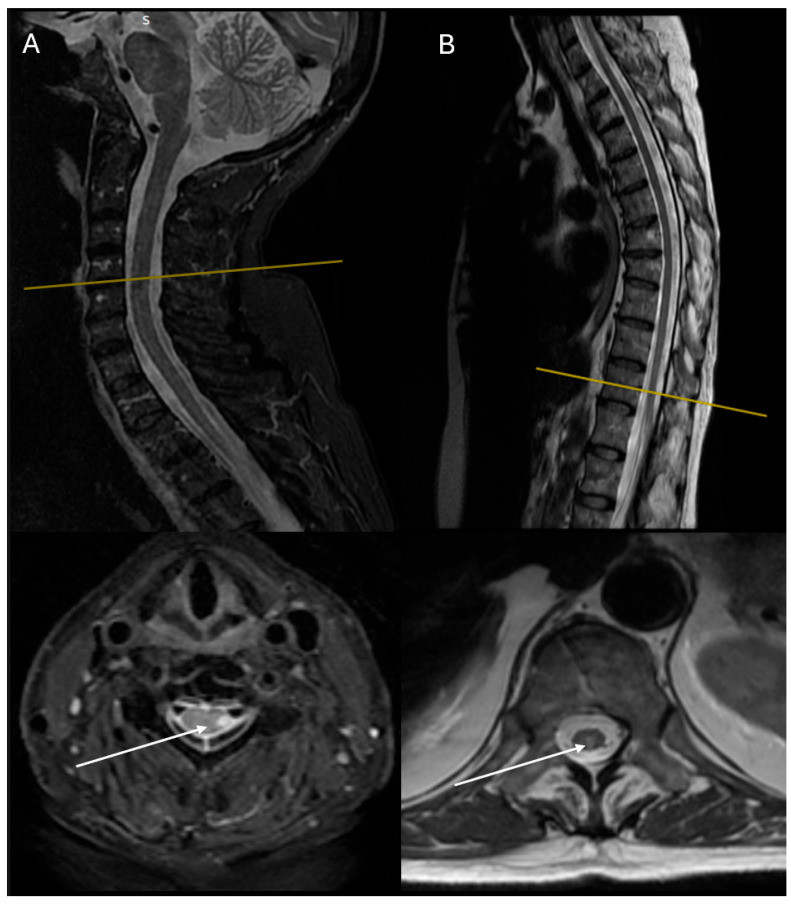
MRI imaging of the second case demonstrating a long segment of a well-defined lesion extending from C3 to C7, appearing low on T1 with no gadolinium enhancement and high on T2: (A) sagittal and axial T2 STIR-weighted MRI images of the cervical spine at admission; (B) sagittal and axial T2-weighted MRI images of the thoracic spine 1 year of follow-up. White arrows show the lesions of hyperintensities involving the dorsolateral part of the cord. One year later, lesions were increased in number but reduced in extent, while T2 hyperintensities were weaker as well.
